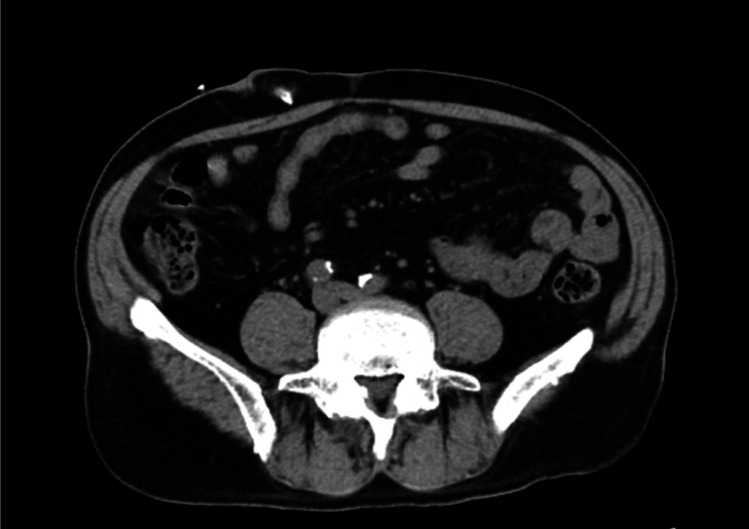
Plain computed tomography (CT) imaging of the first episode of peritonitis
